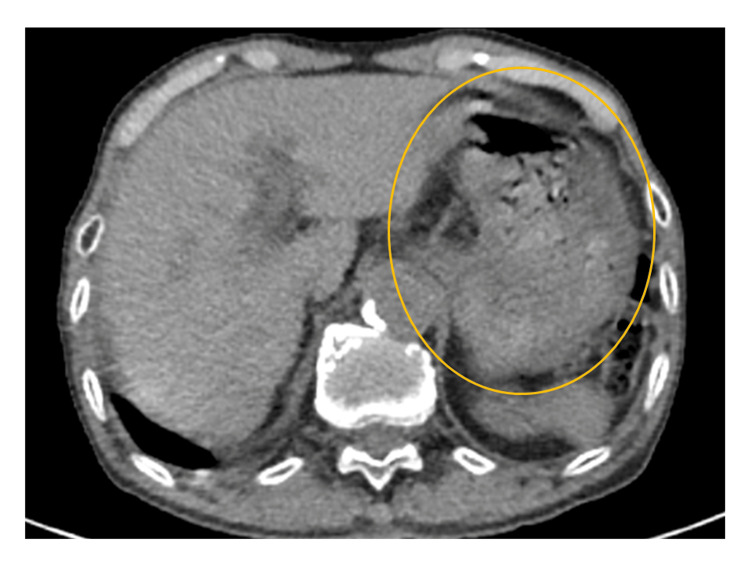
CT scan that was performed concurrently with the PET/CT scan. PET/CT: Positron emission tomography-computed tomography. A large amount of content was found in his stomach (circle).
